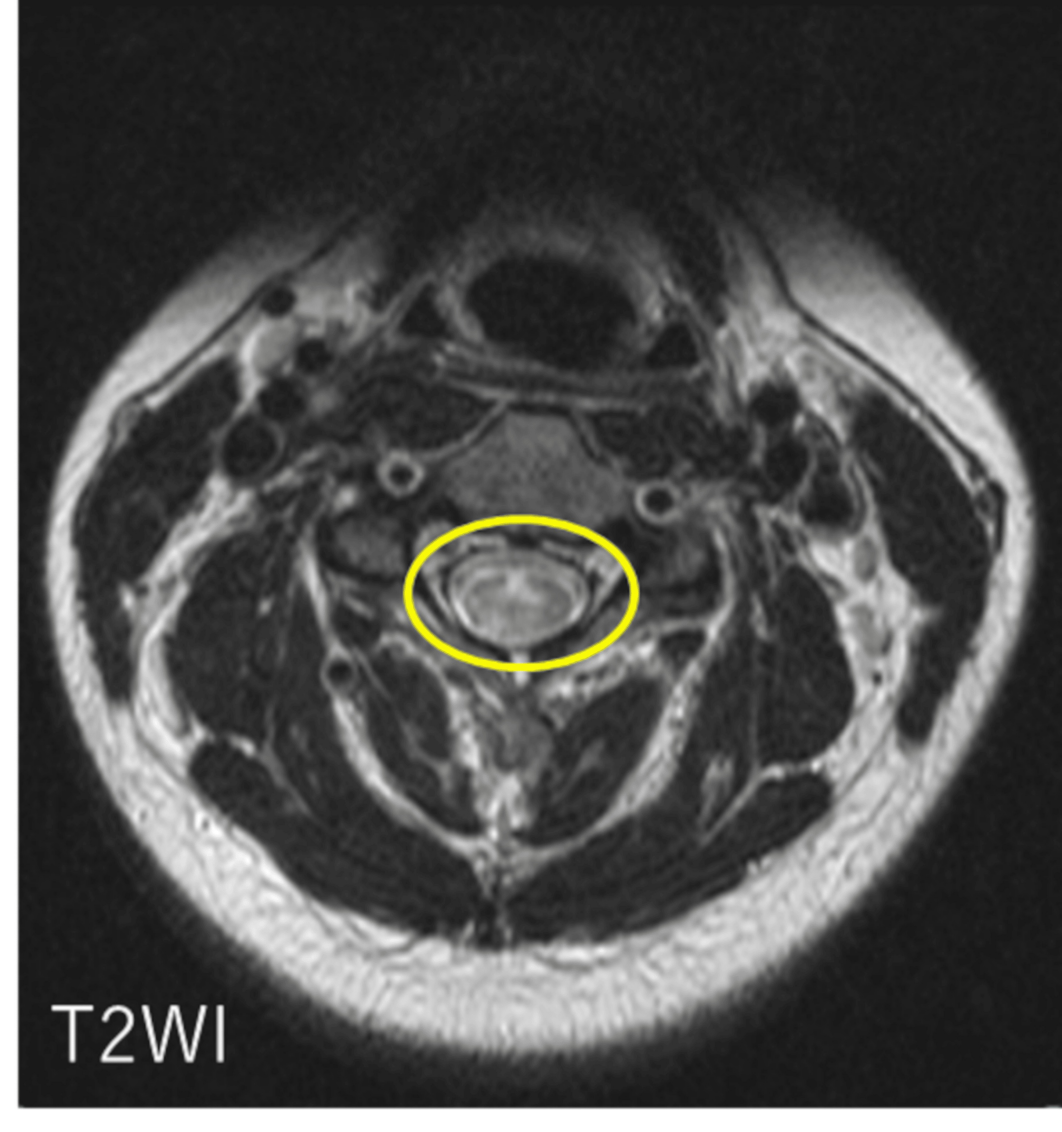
Axial non-contrast MRI of the cervical spine Axial T2-weighted MRI at the C3 level showing right-sided spinal cord hyperintensity (yellow circle).
